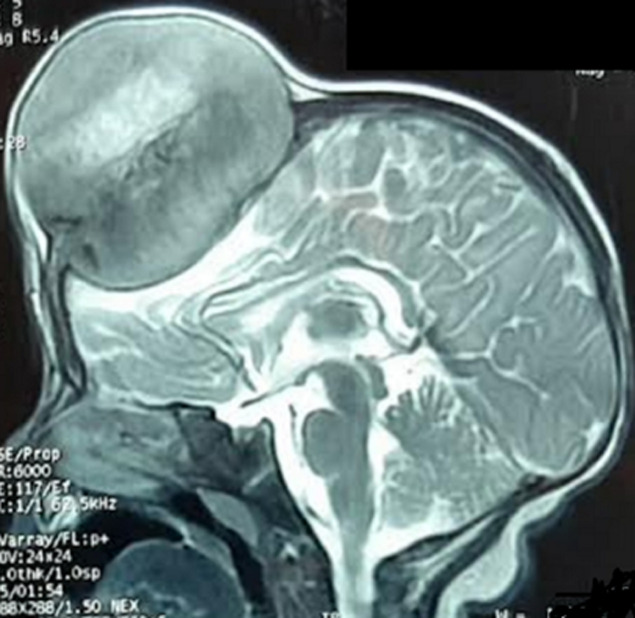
cerebral magnetic resonance imaging sagittal plane in T2-weighted sequence
